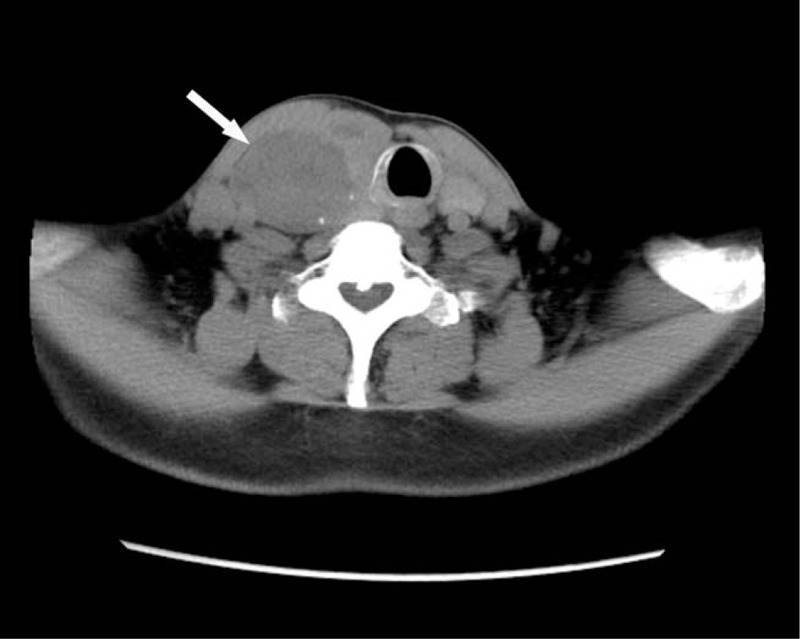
CT image in 2018. Arrow shows the lesion, with sand-like calcification around the lesion.
